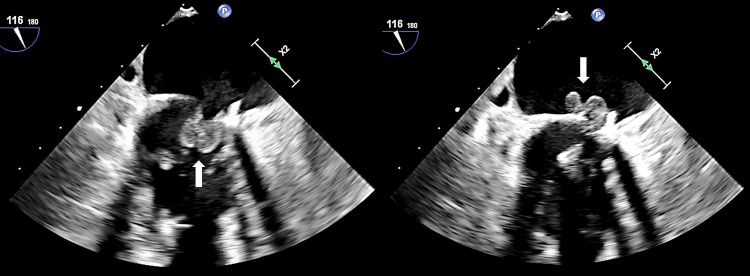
TEE showing 1.6x0.8 cm multi-lobulated vegetation during systole and diastole (arrows). TEE: transesophageal echocardiogram
